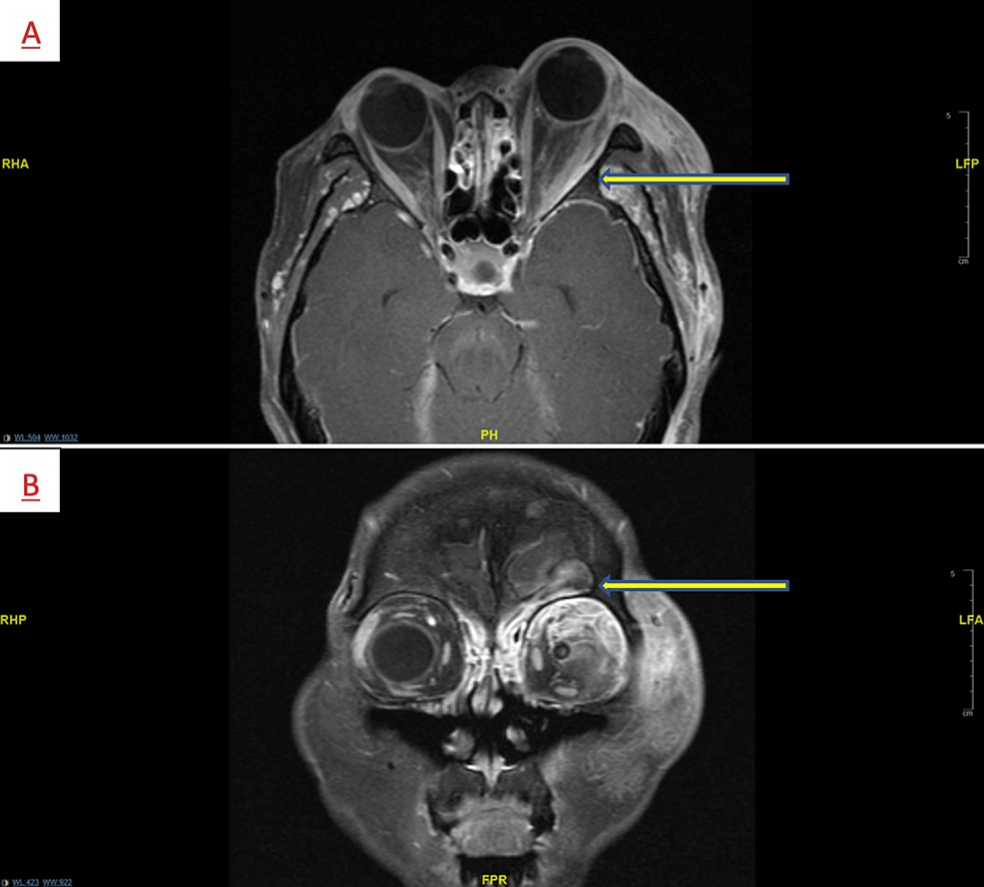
MRI orbits with and without contrast 1A and 1B: There are diffusely thickened superior and lateral rectus muscles of the left orbit. There is an abnormal enhancement along the adjacent roof and lateral walls of the left orbit. However, there is proptosis of the left orbital globe. The left optic nerve is normal. There is preseptal periorbital soft tissue swelling. There is diffusely thickened enhancing dura surrounding the visible frontal lobes bilaterally. The findings are most suspicious for orbital cellulitis in addition to preseptal cellulitis on the left side. A less likely possibility would include metastatic infiltration.
